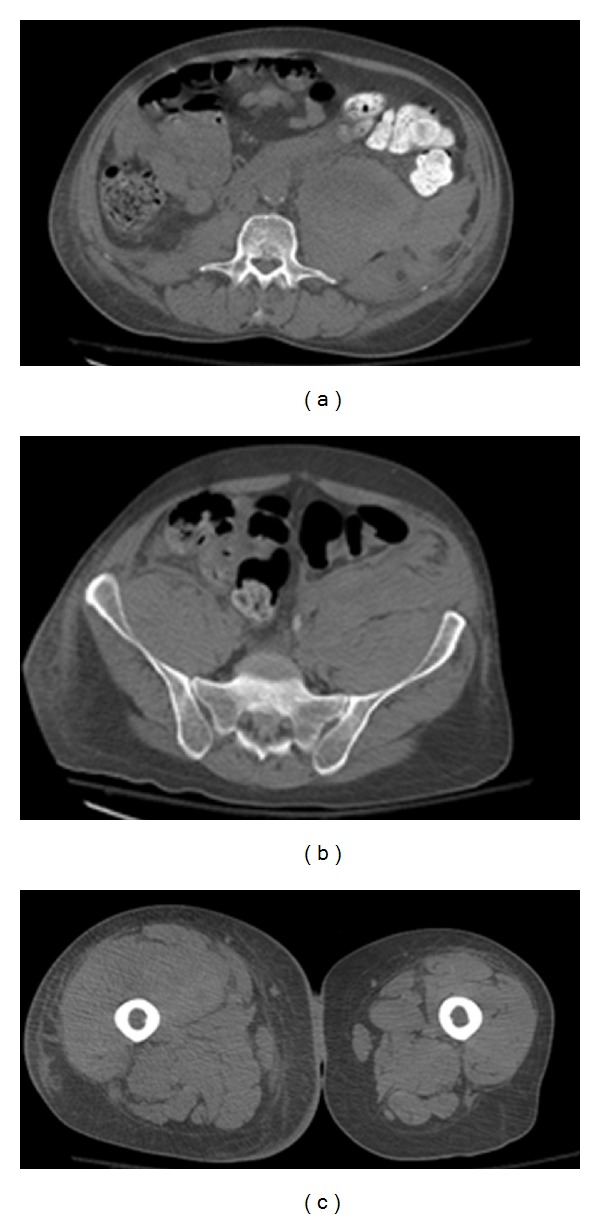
On admission, computed tomography angiogram (CTA) of the abdominal arteries without the use of intravenous contrast revealed large hyperdense retroperitoneal hematomas expanding both psoas muscles, left more than right, that extend into the iliopsoas muscles, and right quadriceps femoris musculature.
